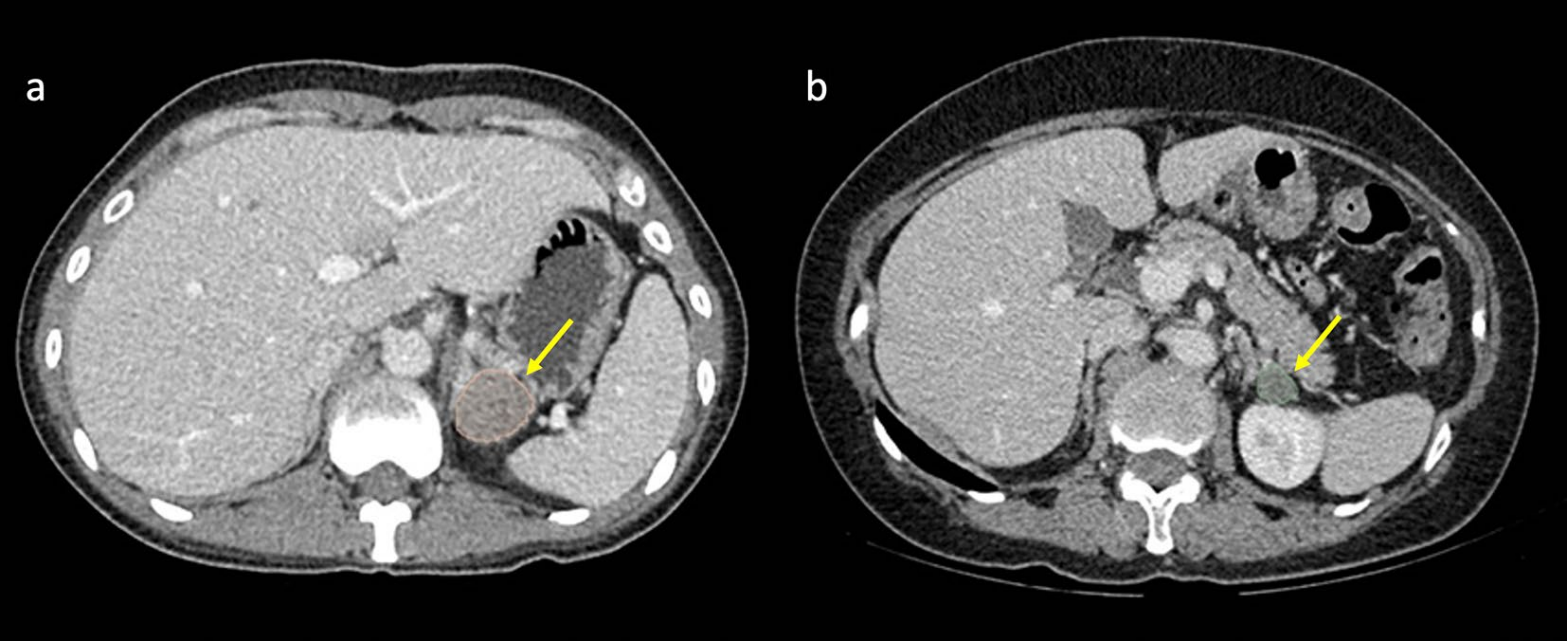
Adrenal nodule segmentation. (a) A 38-year-old female with primary aldosteronism refractory to medical treatment for hypertension. Abdominal CT showed a left adrenal nodule (arrow), and clinical success was achieved after laparoscopic adrenalectomy. (b) A 64-year-old female diagnosed with essential hypertension with a left adrenal nodule (arrow).
